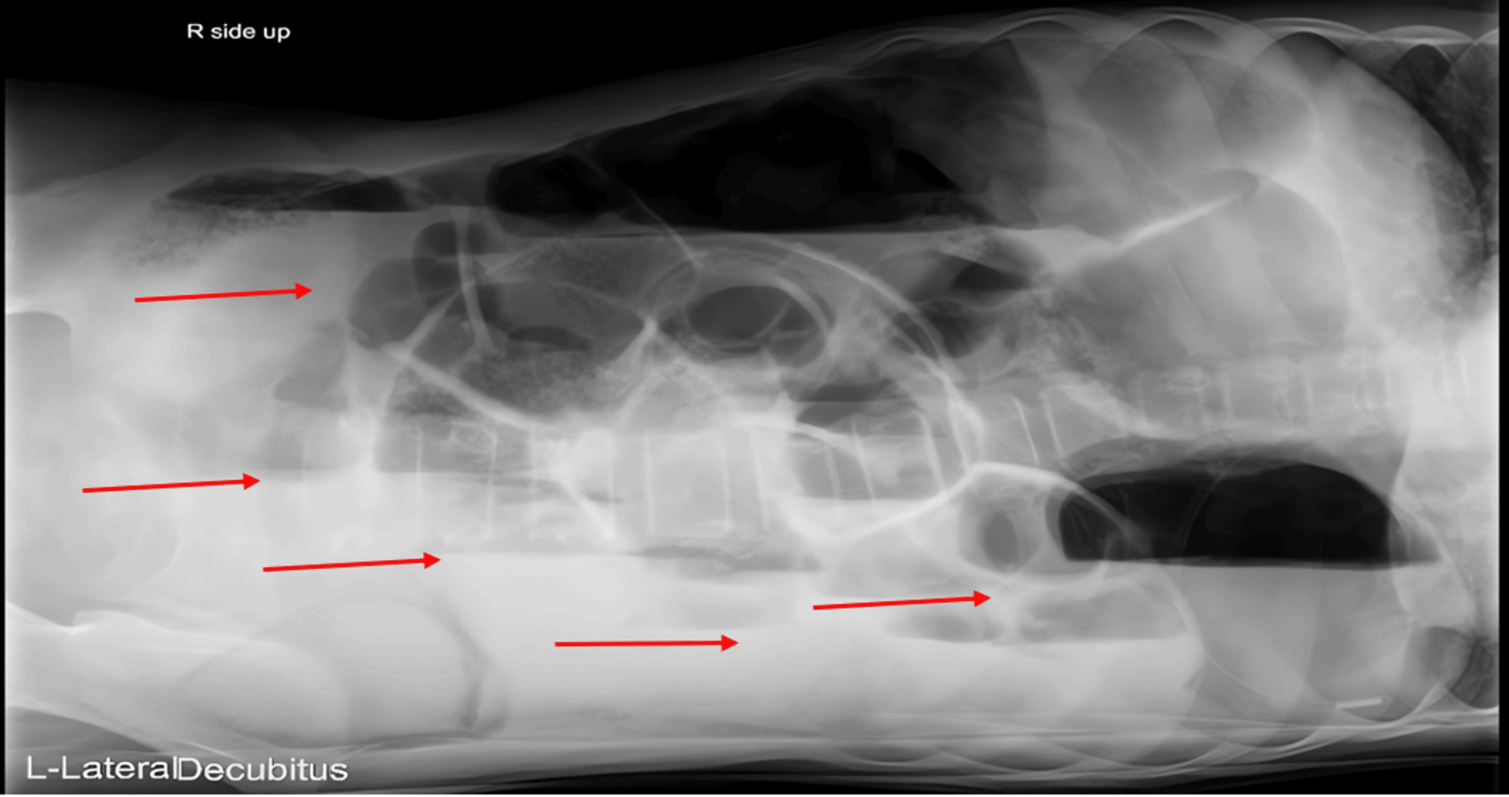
Lateral Decubitus Abdominal Radiograph Initial imaging radiograph revealing significant gaseous distension and multiple air-fluid levels (red arrows).
